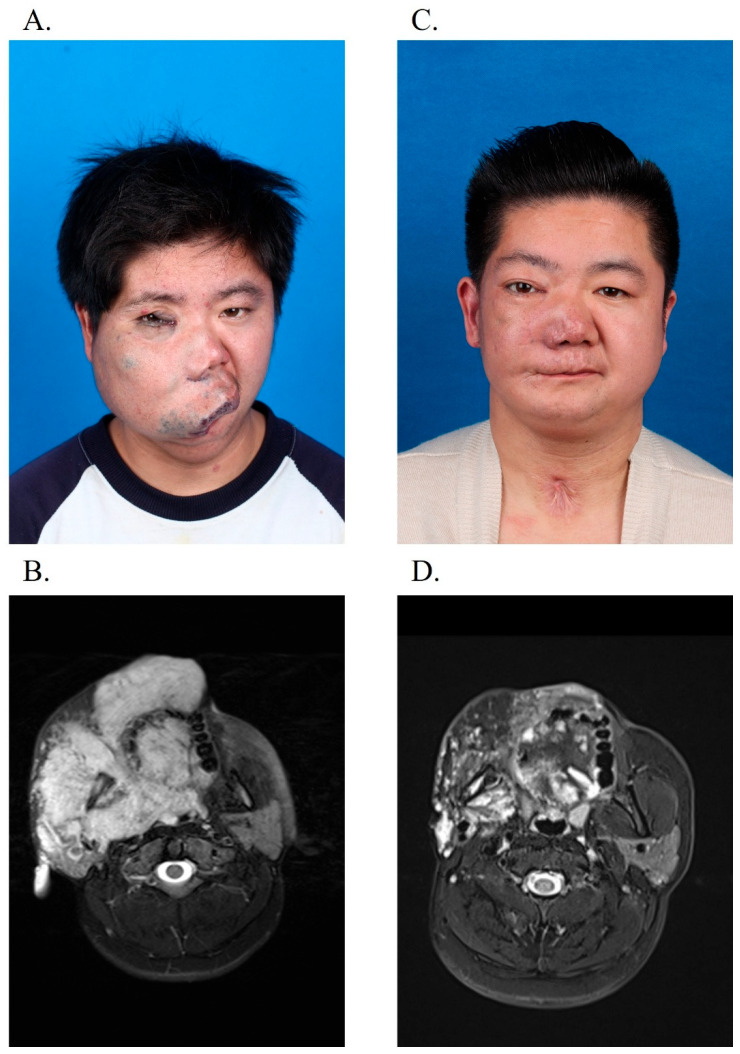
A 23-year-old male suffered from venous malformation involving the right face and neck. (A) Extensive venous malformations involved the right hemifacial and cervical regions. (B) MRI confirmed lesion extension into peripharyngeal tissues, encompassing the hypopharynx and tongue base. (C,D) Post-sclerotherapy and tracheostomy decannulation, 49-month follow-up revealed marked lesion regression.
